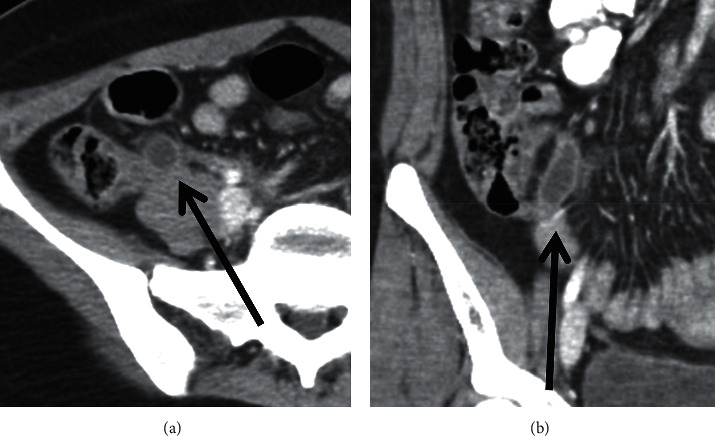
CT scan (transverse and coronal cut). Thickened fluid-filled appendix with localized fat stranding; an appendiceal diverticulum cannot be seen.
